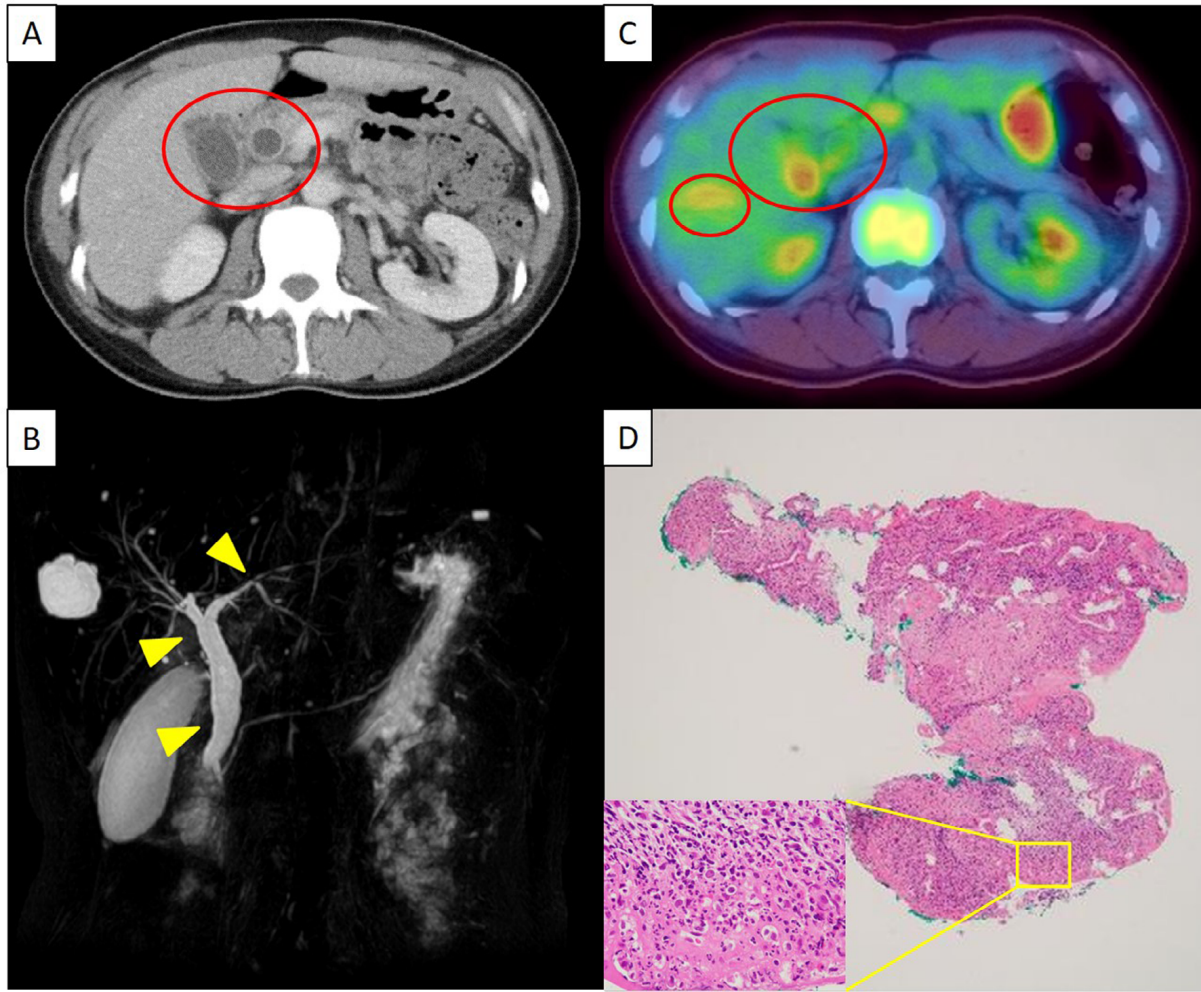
Diagnostic images of a 50‐year‐old Japanese woman diagnosed with lung adenocarcinoma. (A) Enhanced computed tomography (CT) scan revealed oedema‐like thickening of the gallbladder wall, dilation of the bile duct from the common bile duct to the intrahepatic bile ducts, and thickening of the common bile duct wall without any visible obstructions. (B) Magnetic resonance cholangiopancreatography showed irregularly narrowed intrahepatic bile ducts and dilation of peripheral bile ducts. (C) Accumulation of fluorodeoxyglucose (FDG) in the wall of gallbladder and bile duct was also detected with positron emission tomography (PET)‐CT. (D) Biopsy of the extrahepatic bile duct showed numerous neutrophils and lymphoid infiltrates as non‐specific inflammation.
